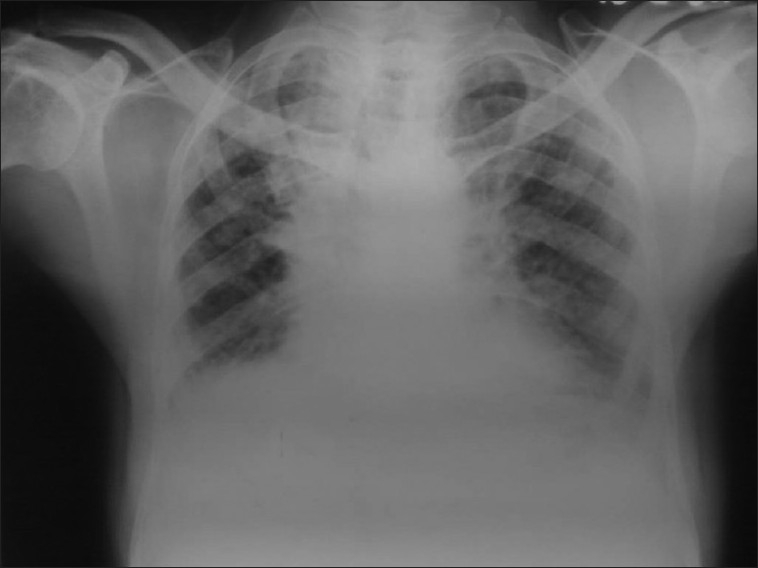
X-ray chest, PA view, after phenytoin withdrawal, showing marked radiological improvement
